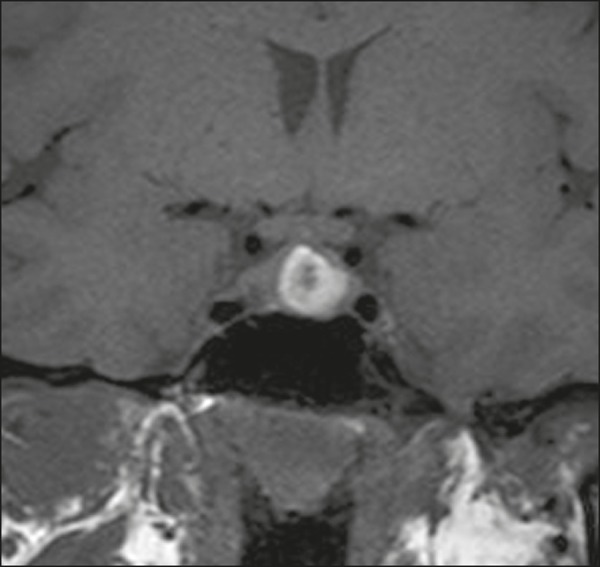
Non-contrast-enhanced coronal T1-weighted MRI sequence showing a
